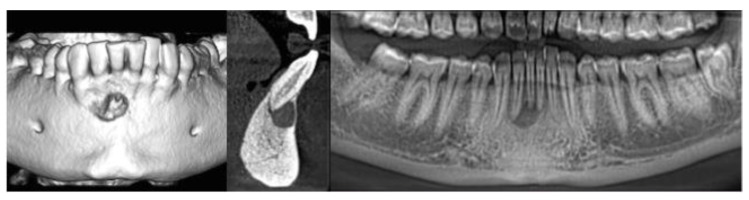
Mandibular periapical cyst in 3D, Sagittal, and Panorex views.
